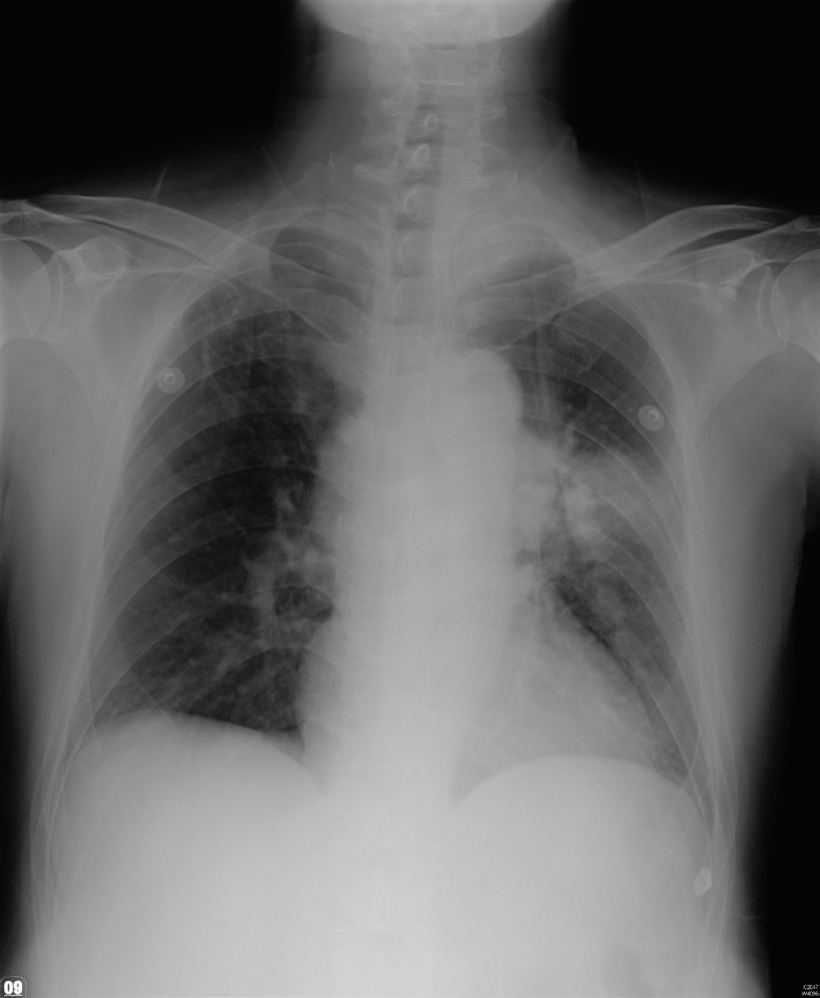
Chest radiography on emergency room shows a soft tissue density mass at left upper lobe with prominent soft tissue density at left apex and left lower neck.
